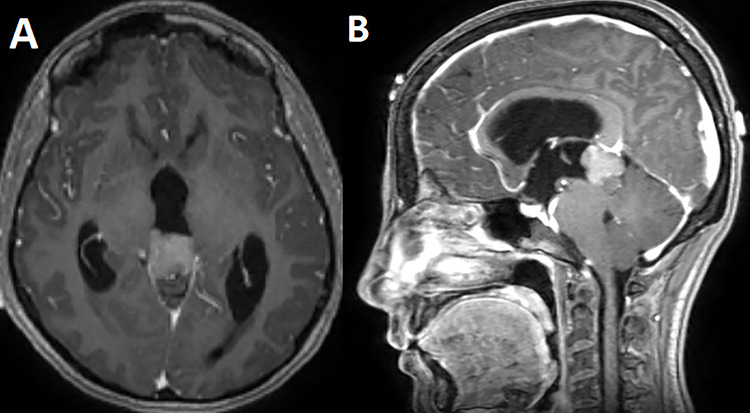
T1 enhanced axial (A) and sagittal (B) MRI showing a T1 hypointense homogenously enhancing pineal tumor.
